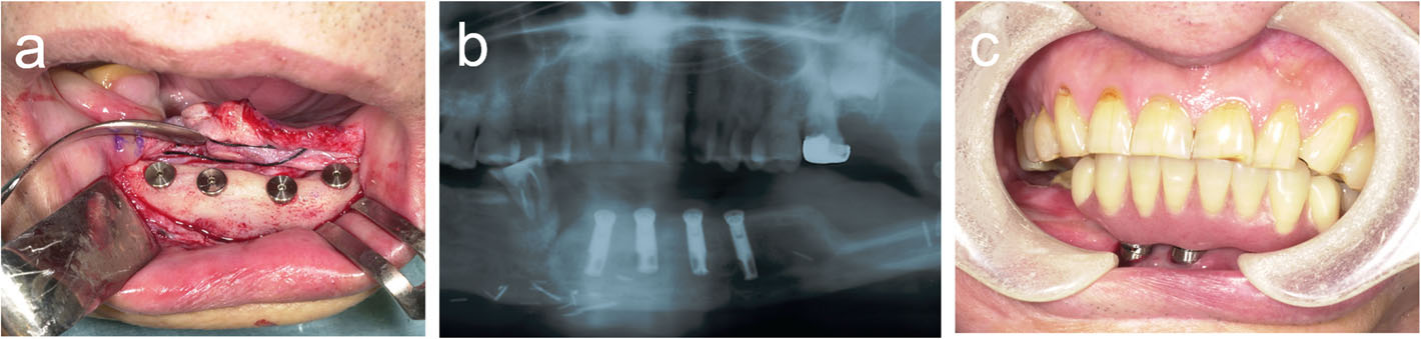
a Four dental implants were inserted into the distracted fibula. b Orthopantomogram after implantation. c Intraoral view after treatment: the implant denture was placed on the mandible
